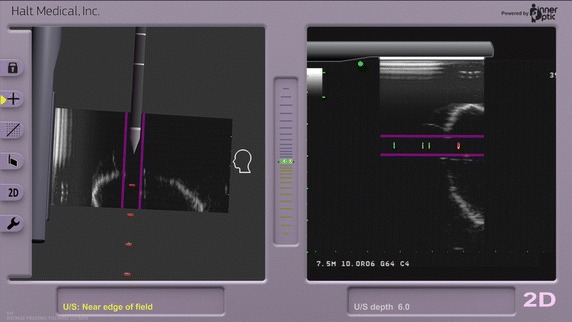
Acessa Guidance screens. (*Left*) Laparoscopic ultrasound view of Acessa handpiece tip entering fibroid from left to right within the two (*purple*) virtual tracking lines of the target zone; (*right)* computer-generated view of the relationship of the ultrasound beam and trajectory of the handpiece tip into uterus and fibroid
